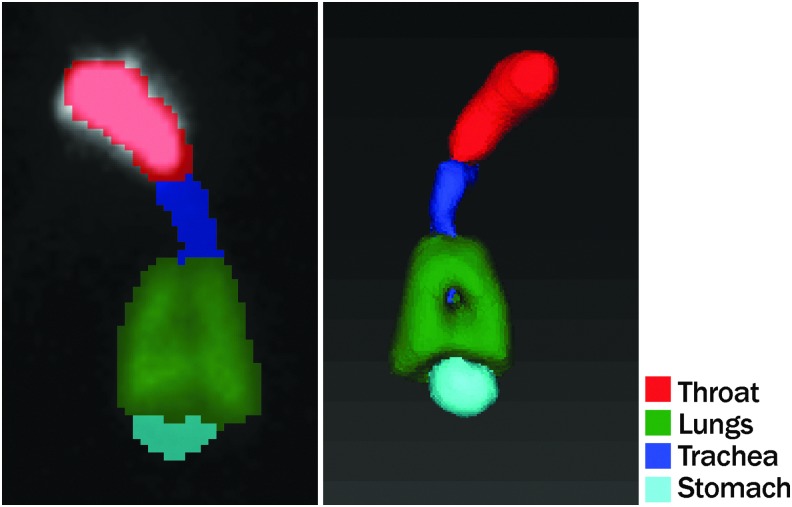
Typical region of interests for planar (left) and SPECT (right) image analysis. SPECT, single-photon emission computed tomography.
